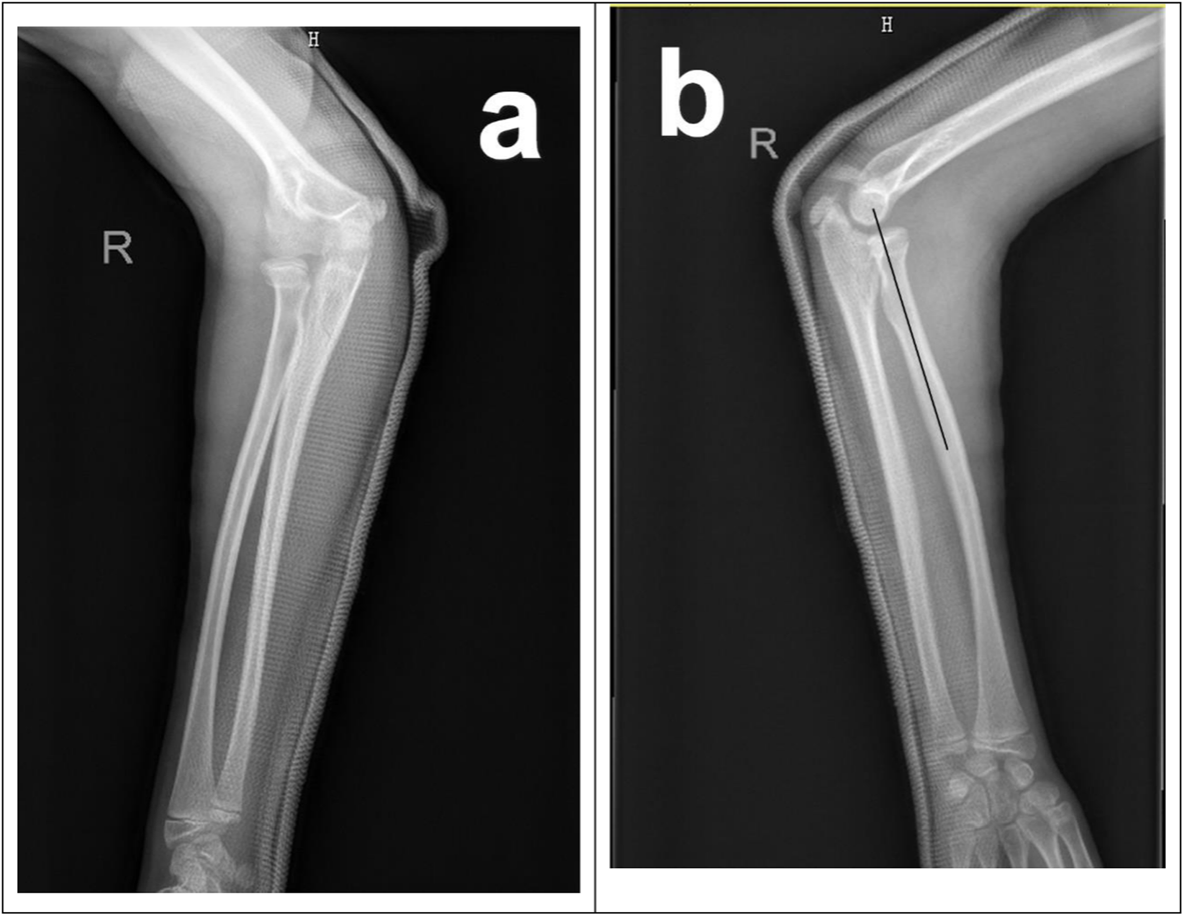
a AP X-rays showing proper fracture and joint position after initial closed reduction. b Lateral X-rays showing proper fracture and joint position after initial closed reduction. Black line showing axis of radius, the extension of which went right through the capitellum
